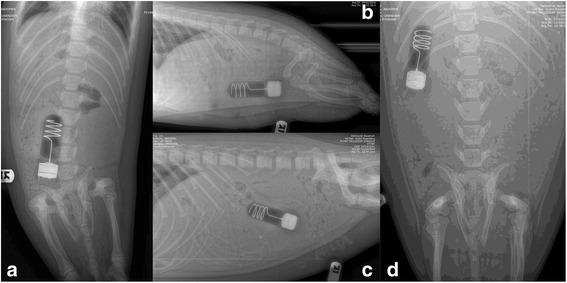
Abdominal radiographs of female harbor seal pup Pv14–062. Immediately after surgery (a: dorsoventral, b: lateral view) the LHX tag (98 mm length × 33 mm diameter) has a relative length of about 4 vertebrae. 78 days after surgery (c: lateral, d: dorsoventral view) the LHX tag has a relative length of about 3 vertebrae
